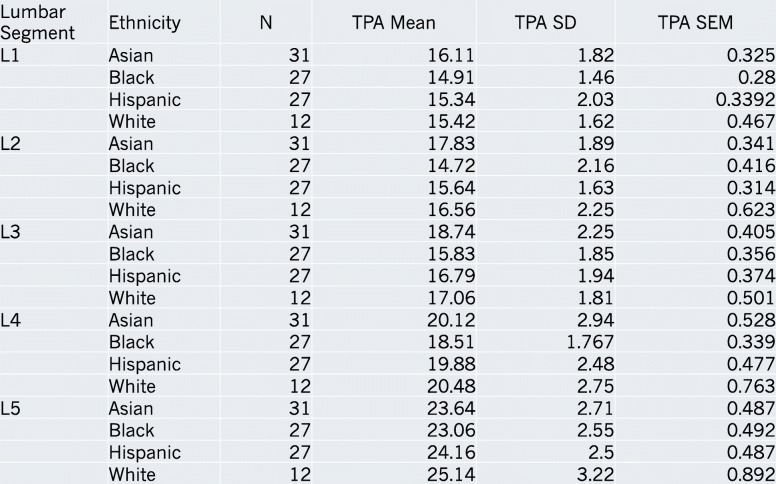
Mean TPA Data. Data obtained via CT analysis of mean TPA, standard deviation and standard error of the mean classified by race and individual lumbar level
